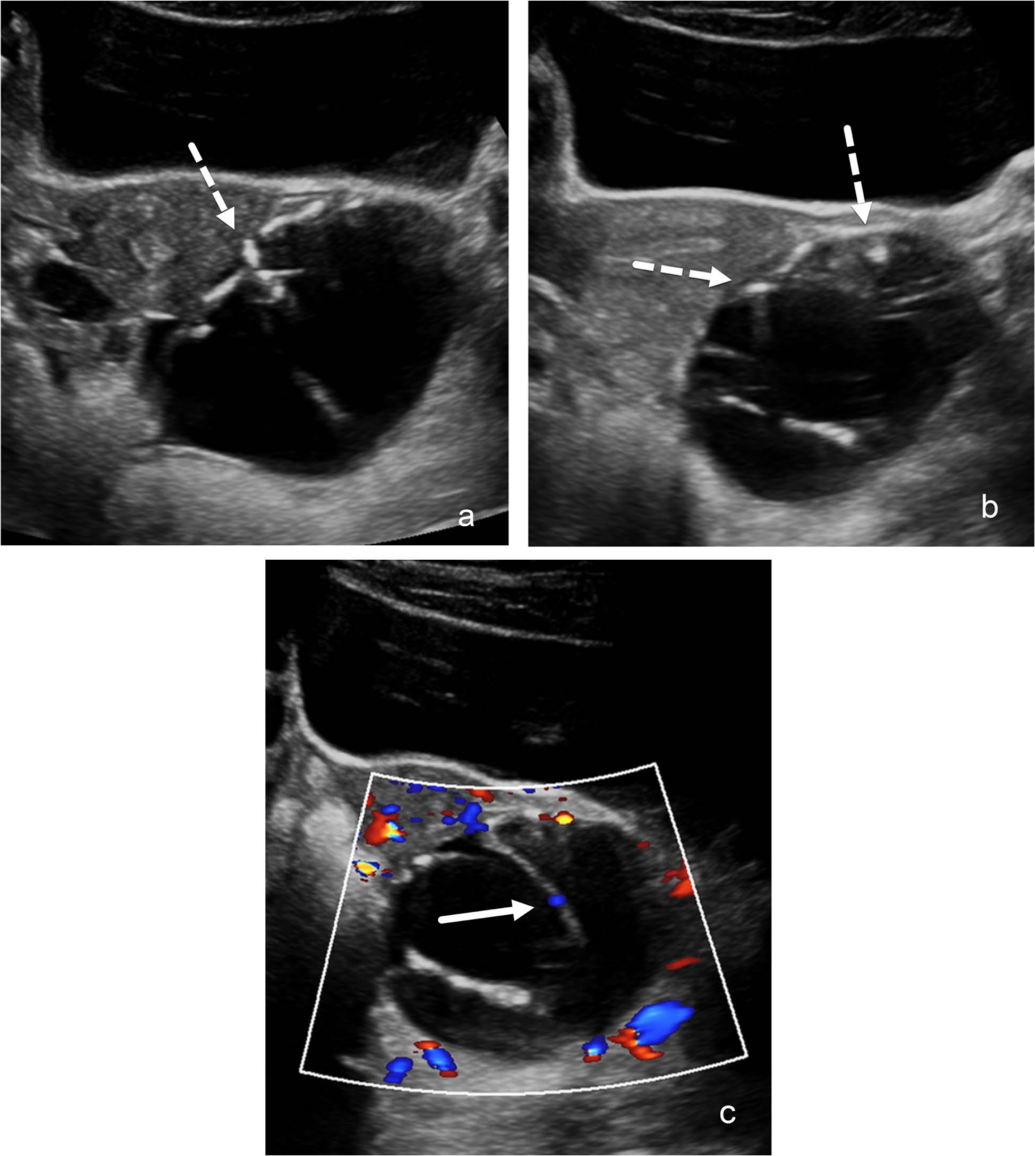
Ultrasound image example of a false positive ovarian/adnexal lesion which all 3 readers labeled incorrectly in a 16-year-old female: Transverse static grayscale (a, b) and color Doppler (c) ultrasound images demonstrates a 7.4-cm complex multilocular cystic lesion of the left ovary, with several internal septations measuring up to 4 mm. Inner walls and septations demonstrate irregular contours, with scattered echogenic foci/calcification (*dashed arrows* in a and b). No solid components are identified, although there is minimal flow (color score, CS=2) seen on the color Doppler image along a few septations (*solid arrow *in c). All three reviewers labeled this lesion as O-RADS 4 that was pathologically proven to represent a mucinous cystadenoma
